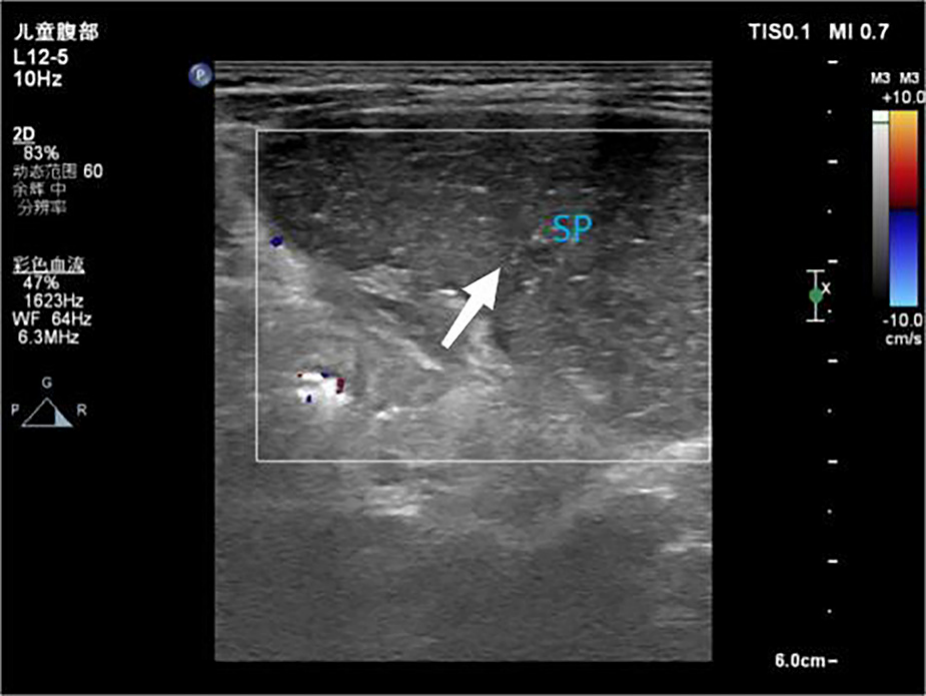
Ultrasound image showing no blood flow signal within the spleen.
